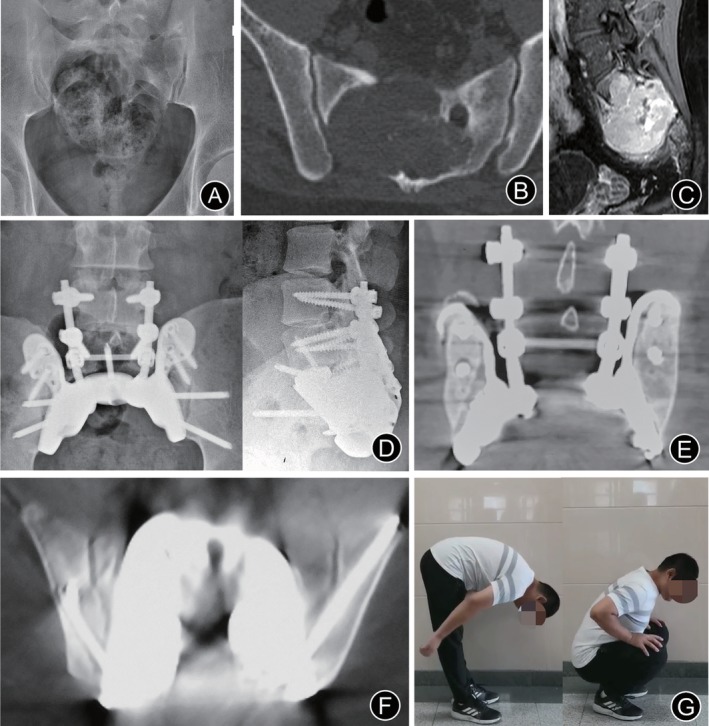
Imaging of the representative case. Preoperative X‐ray (A), axial CT (B), and contrast‐enhanced sagittal MRI (C) revealed a large soft tissue mass located in the upper sacrum. Postoperative X‐ray (D), and coronal (E) and axial (F) CT showed good implant alignment, no evidence of implant loosening, excellent bone ingrowth, and osseointegration at the bone–implant junctions in the 17 months after surgery. (F) He could squat and bend like a normal person.
